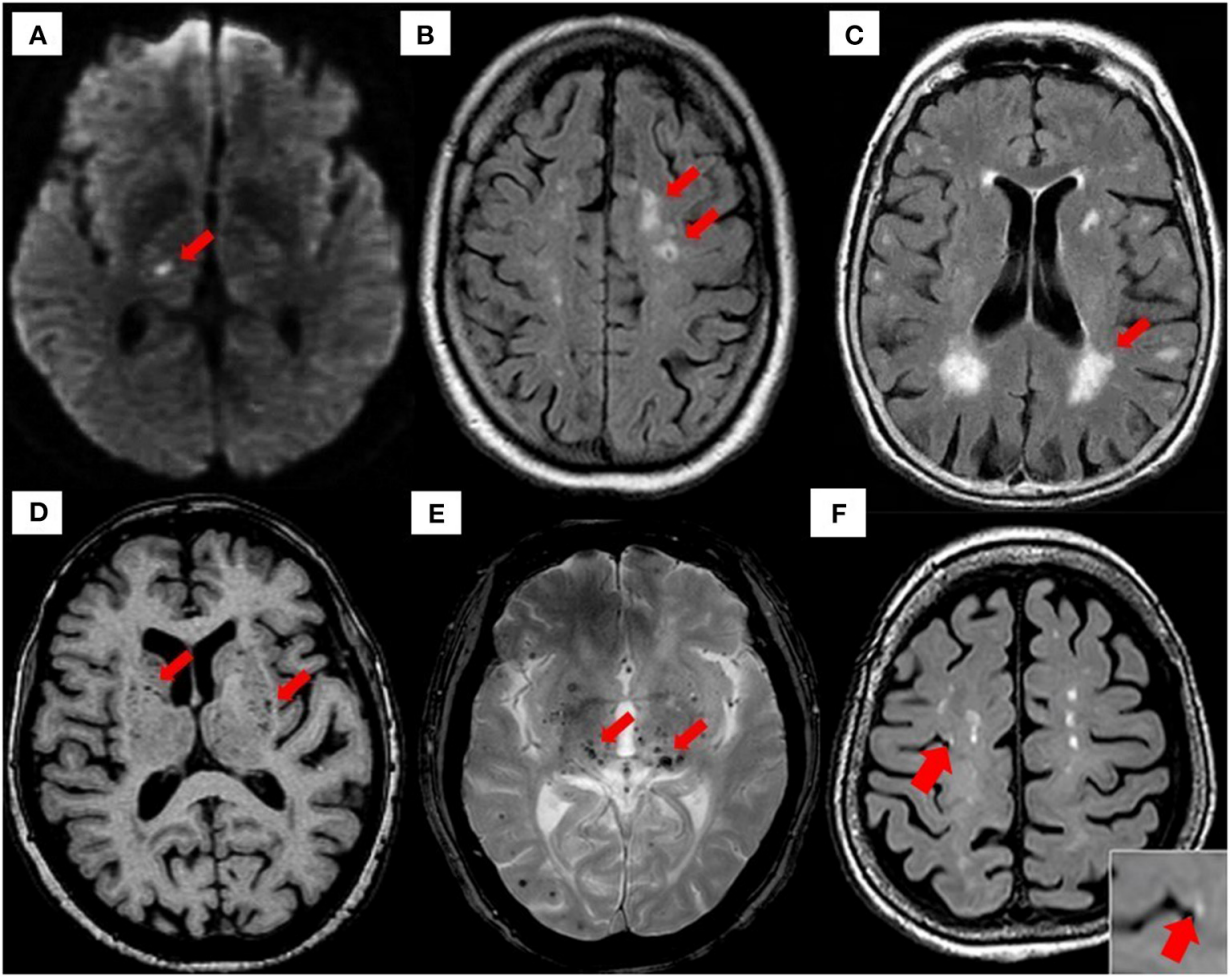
Neuroimaging correlates of CSVD based on STRIVE method. (A) Recent small subcortical infarct (RSBI) on diffusion-weighted imaging (DWI) (red arrow). Usual diameter is around 3–15 mm, with hyperintense rim surrounding ovoid cavity. RSBI seen as increased T2-weighted, fluid-attenuated inverse recovery (FLAIR), and DWI signal intensities decreased T1-weighted signal and isointense in T2*-weighted gradient recoiled echo (GRE) signal and susceptibility-weighted imaging (SWI). RSBI is best identified through DWI with usual infarct diameter of ≤20 mm. (B) Lacunar infracts on FLAIR (red arrow). Lacunar infarcts appeared as increased hyperintensity in T2-weighted signal, decrease T1-weighted, and FLAIR signal and isointense in DWI. Usual diameter is around 3–15 mm, with hyperintense rim surrounding ovoid cavity. (C) White matter hyperintensities (WMHs) of presumed vascular origin on FLAIR (arrow). WMHS seen as increase intensity or hyperintensity on T2-weighted imaging, T2*-weighted GRE and FLAIR (best identified), isointense on DWI, and hypointense (decrease intensity) on T1-weighted imaging. (D) Enlarged perivascular spaces (PVS) on T1-weighted imaging (red arrow) with usual diameter of ≤2 mm. PVS is seen as decrease FLAIR and T1-weighted signal intensity, with increase T2-weighted signal. Meanwhile T2*-weighted GRE and DWI appeared isointense, and they also appeared in similar signal intensity with cerebrospinal fluid (CSF). (E) Cerebral microbleeds (CMBs) on T2*-GRE (red arrow). CMBs are small, rounded areas of signal void with blooming, whereby they were visualized as isointense T1- and T2-weighted signal, FLAIR, and DWI. They are best identified under T2*-weighted GRE or SWI as reduced signal intensities. Usual diameter is around ≤10 mm (mostly 2–5 mm). (F) 3-T MRI representation of cortical microinfarcts (red arrow) on T1-weighted (hypointense) [images A–E, reproduced with permission from Mustapha et al. (57), image F is adapted from Takasugi et al. (61)].
